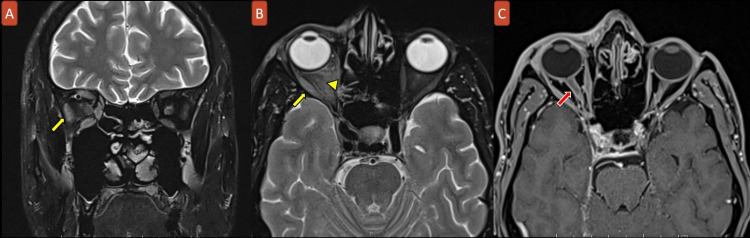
MRI brain with fat saturation in coronal T2WI (A) and axial T2WI (B). Axial T1WI with contrast (C) showing mild diffuse enlargement of the extraocular muscles in the right orbit with increased enhancement (yellow arrows) in addition to diffuse optic nerve sheath enhancement in the right orbit (red arrow) with mild retrobulbar orbital fat haziness (yellow arrowhead) indicating orbital myositis and preoptic neuritis.
